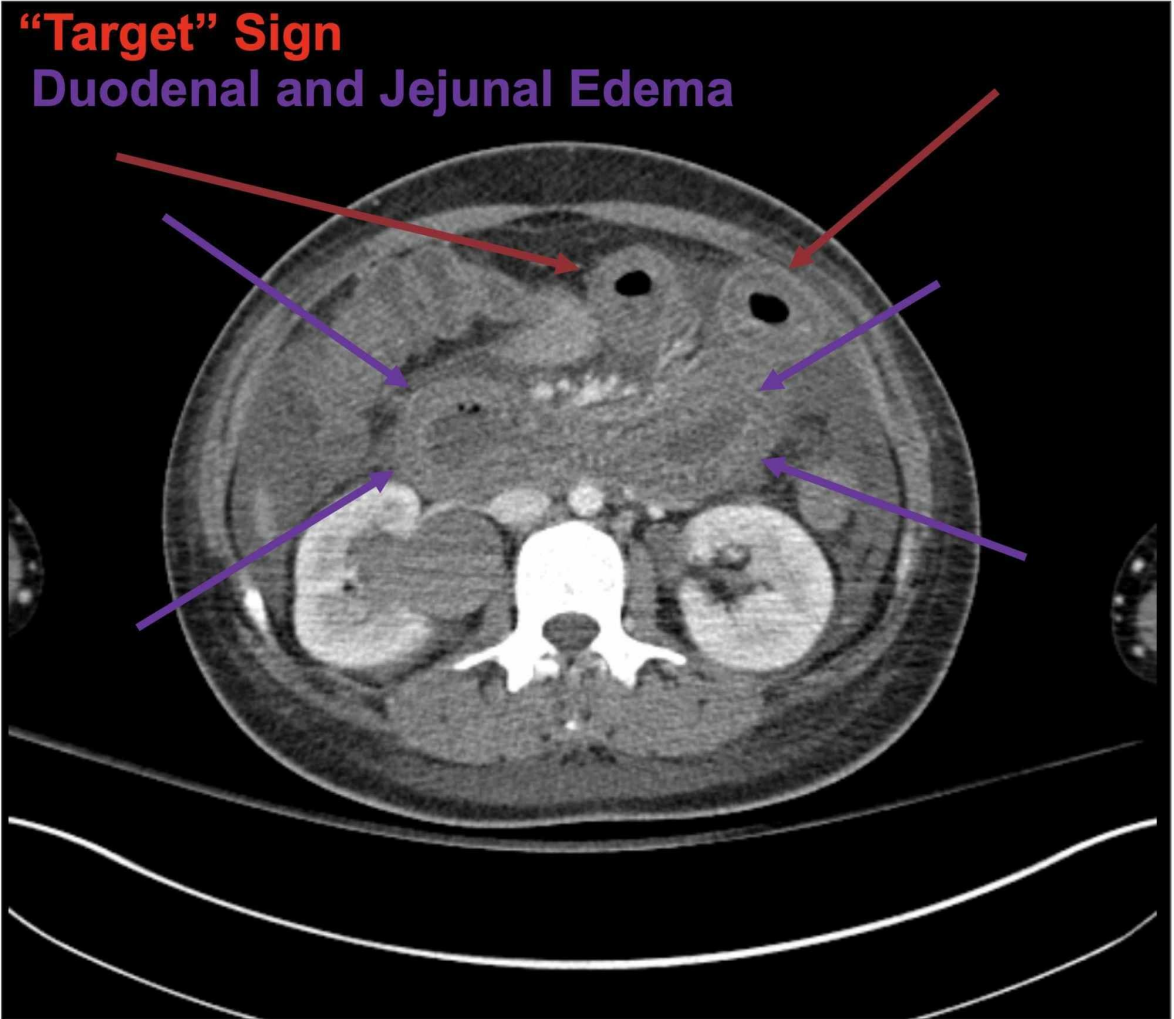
CT scan of the abdomen and pelvis with IV contrast Jejunum (purple arrows); duodenum (red arrows)
